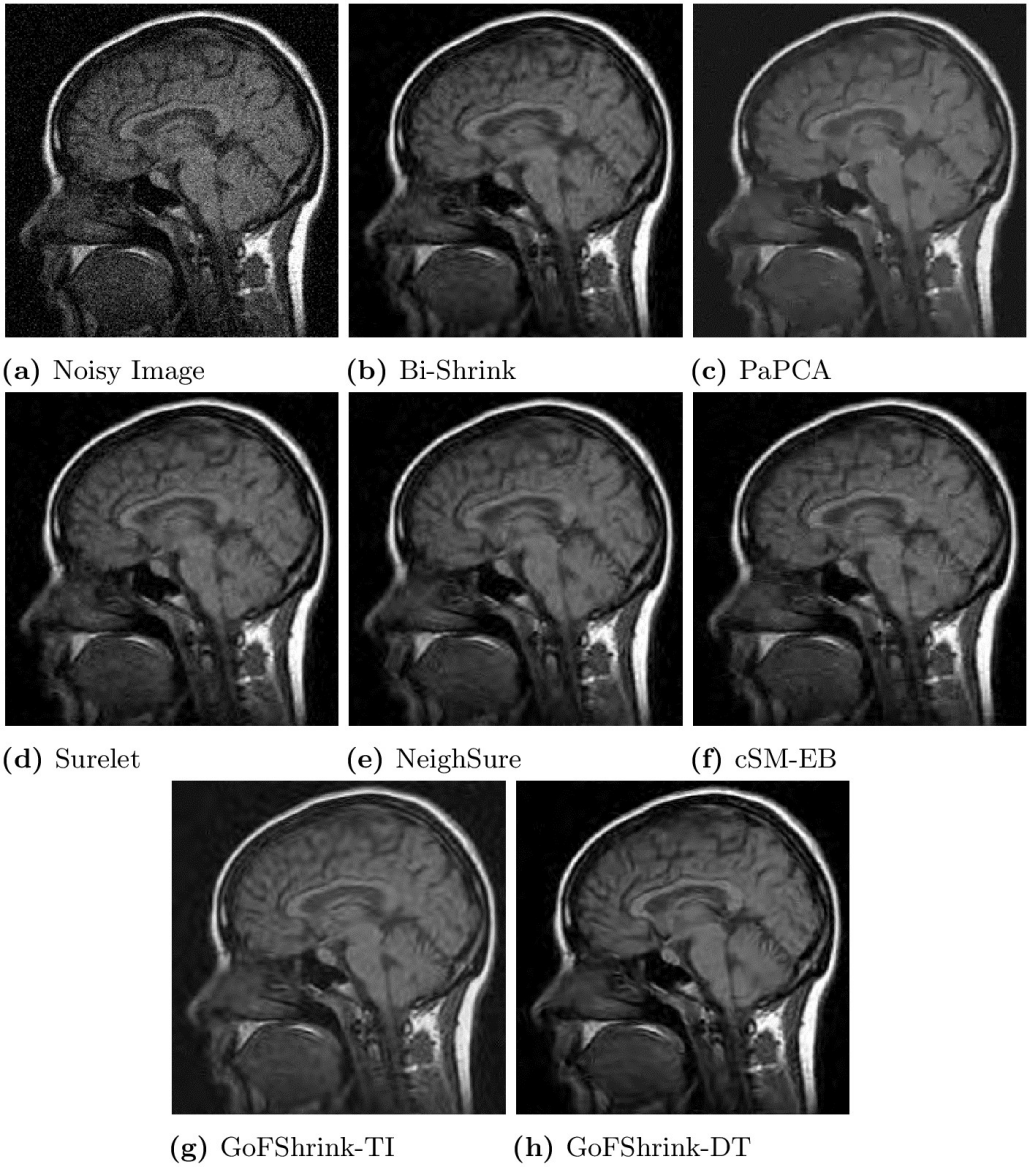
Visual results for several state-of-the-art image denoising methods on the *Side MRI image* of a brain corrupted with the noise level *σ* = 20. This figure is composed of (a) noisy image; (b) denoised image from *Bi-Shrink*; (c) *PaPCA*; (d) *Surelet*; (e) *NieghSure*; (f) *cSM-EB*; (g) *GoFShrink-TI*; and (h) *GoFShrink-DT*.
